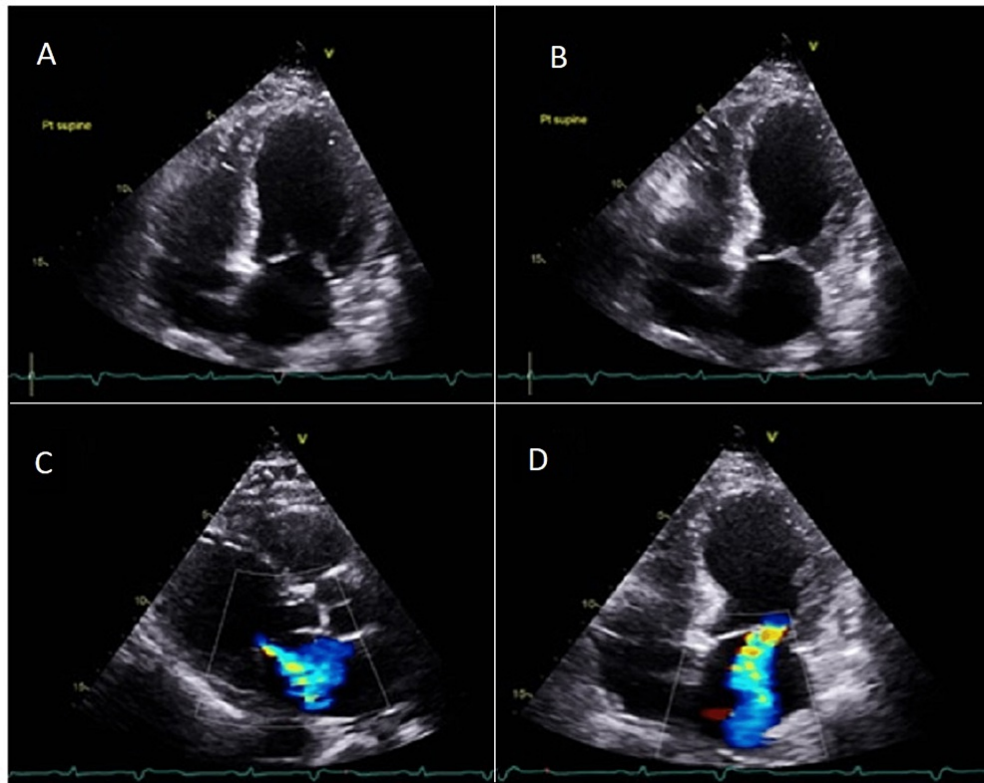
Transthoracic echocardiogram apical four-chamber view in diastolic (A) and systolic (B) period and parasternal (C) and four-chamber apical view (D) demonstrating moderate mitral regurgitation and global hypokinesis.
